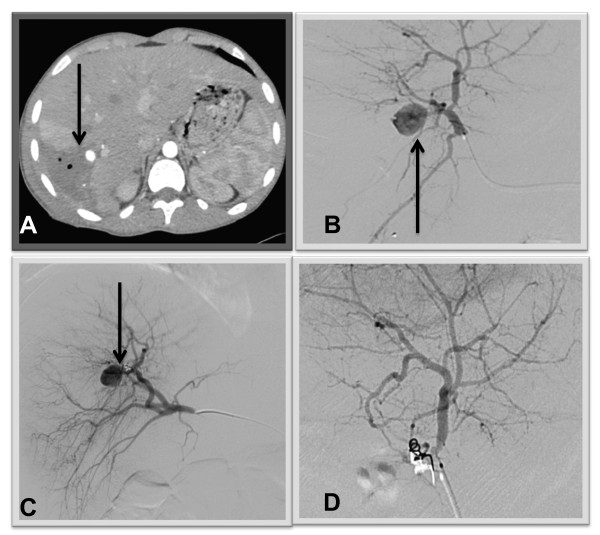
16 year old male sustained a gunshot wound to the right upper abdomen. He was managed non-operatively. Right hepatic artery pseudoaneurysm (PSA) was found on CT performed due to fever, billious discharge from the exit wound and pain on PID 16. Two days after successful AE he had an episode of external bleeding from the same bullet wound. Recurrent PSA was found on repeat angiography. Repeat AE was performed successfully. A: Contrast-enhanced CT scan obtained on PID 16 shows a newly developed, well-circumscribed PSA (arrow) within the perihepatic abscess containing air bubbles. B: On selective arteriogram, the PSA (arrow) is seen to arise from the right hepatic artery. C: Recurrent PSA found 2 days after previous AE. Coils are seen in the artery (arrow). D: Angiogram after successful AE.
